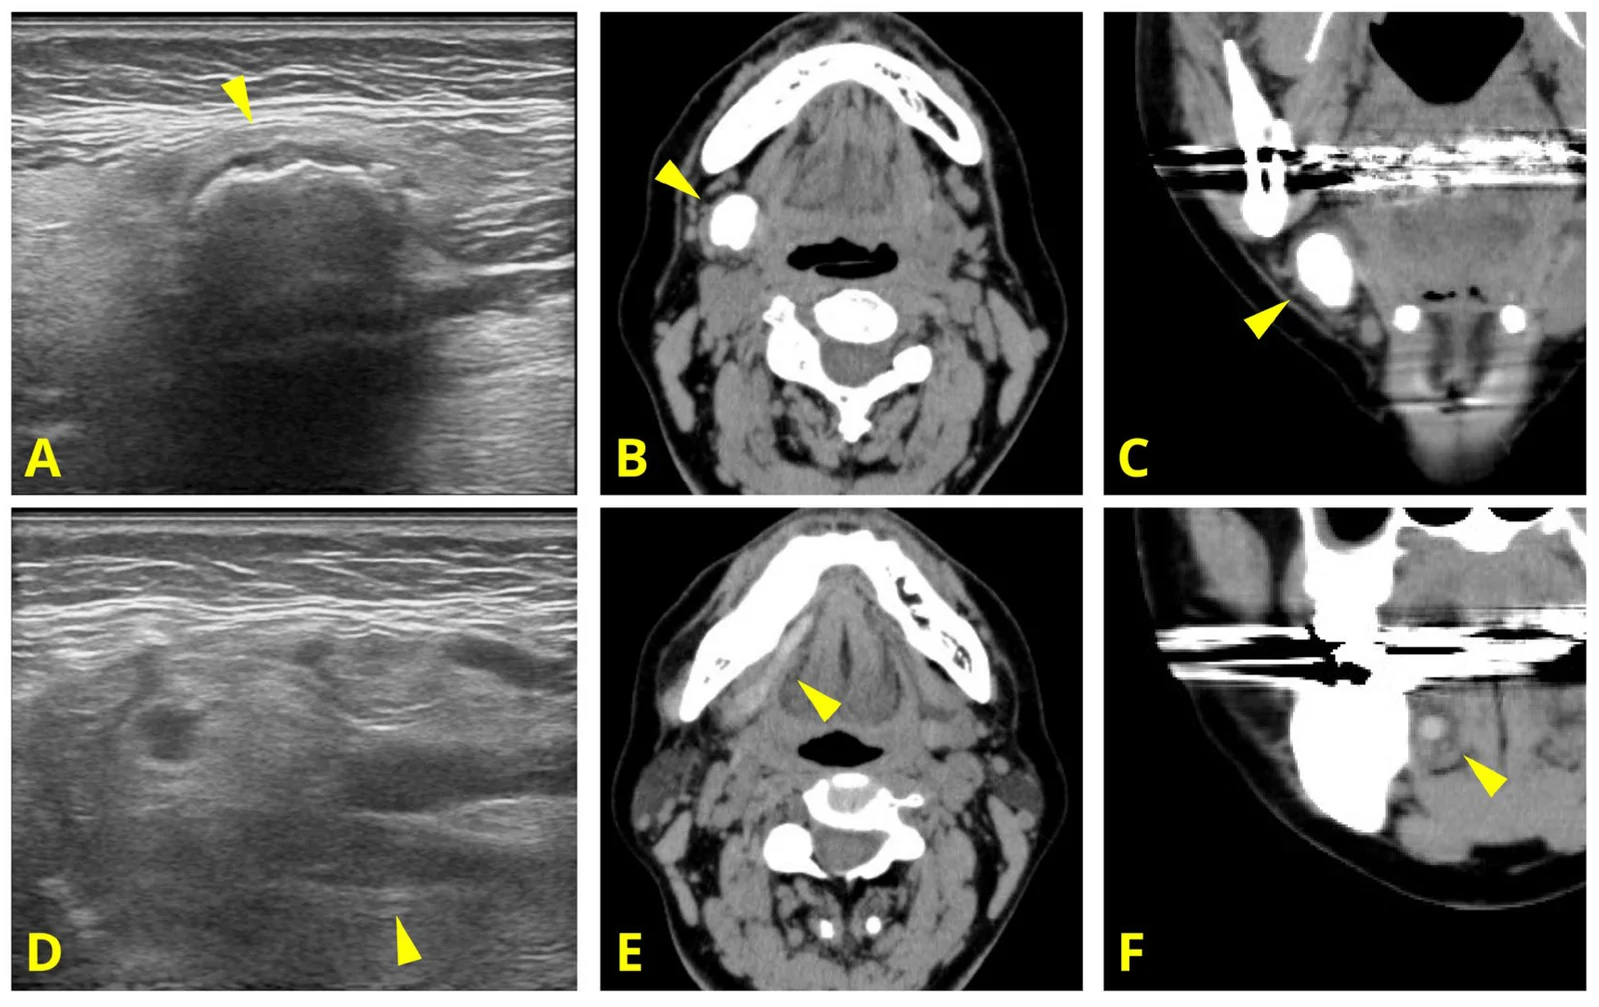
Computed tomography (CT) and ultrasonography findings before right submandibular gland excision: (A,B) A calcified lesion measuring 11 × 14 × 16 mm was observed in the right submandibular gland (arrowheads). (C) A calcified lesion with acoustic shadowing was observed in the right submandibular gland (arrowhead). (D,E) The right Wharton’s duct was dilated, and a high-attenuation area, with a mean CT value of 110 HU, was observed along the duct lumen. Edematous changes were present in the tissue surrounding Wharton’s duct (arrowhead). (F) Dilatation of the right Wharton’s duct was observed. No apparent calcification was detected within the duct (arrowhead).
